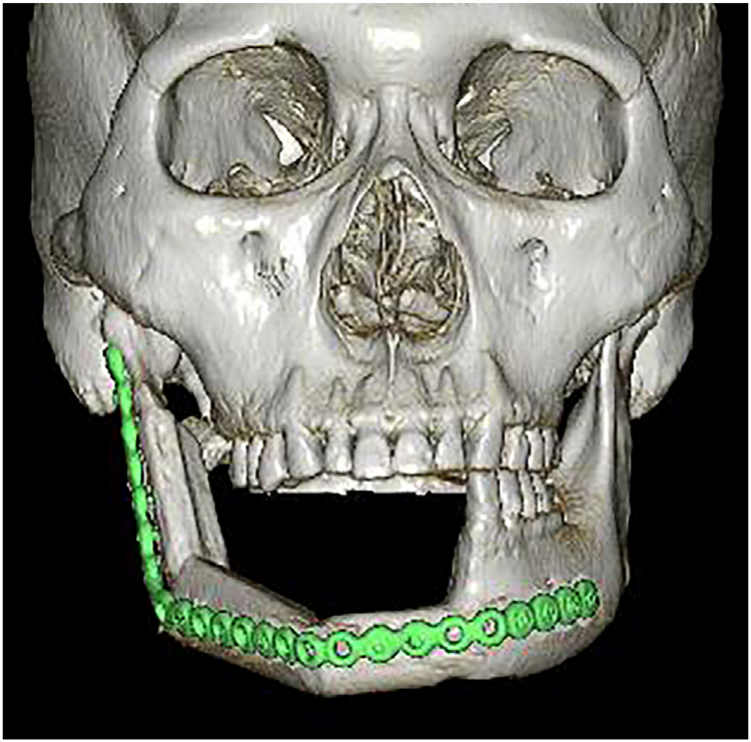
3D-CT at 1 year postoperatively. The reconstructed mandibular morphology is good. 3D-CT, three-dimensional computed tomography.
